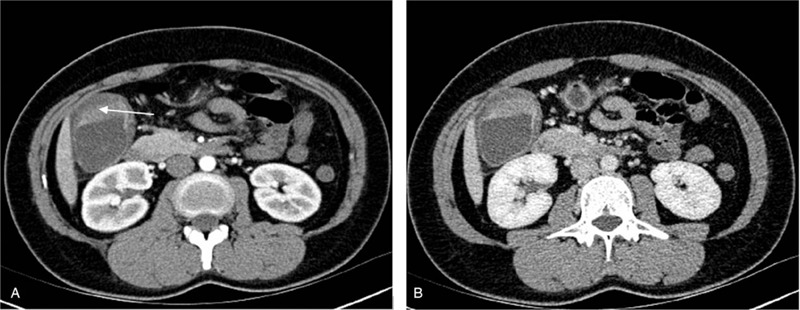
Typical contrast-enhanced CT images for GB-NEC. Continuous thin line-like contrast enhancement on the mucosa at (A) arterial and (B) venous phases. The arrow shows local wall thickening at the bottom of the gallbladder. CT = computed tomography, GB-NEC = gallbladder neuroendocrine carcinoma.
